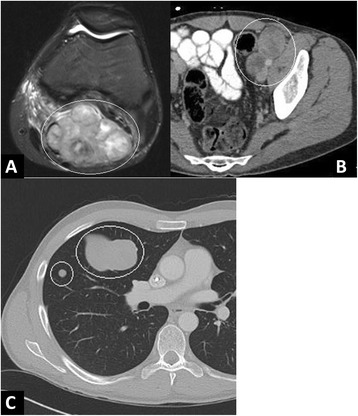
Radiologic presentation. Upon initial presentation, (a) axial MRI (short tau inversion recovery/STIR) demonstrate a large lobulated soft tissue mass within the popliteal fossa, (b) axial contrast-enhanced CT images demonstrate bulky, necrotic left external iliac lymph nodes, and (c) axial CT images demonstrate a dominant *right* lung mass and small nodule consistent with pulmonary metastases
